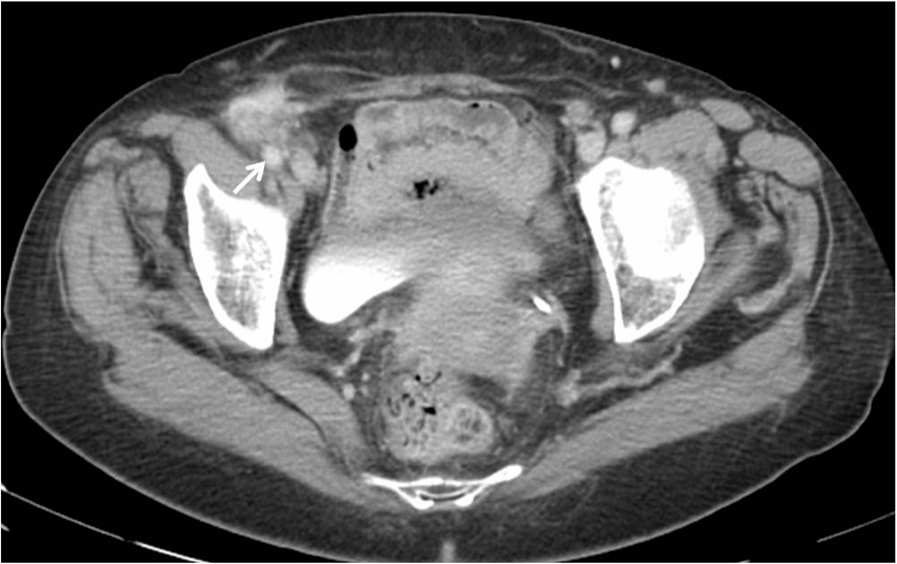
Computed tomography scan shows the cecal wall thickness within a right inguinal hernia involving the femoral artery (arrow).
